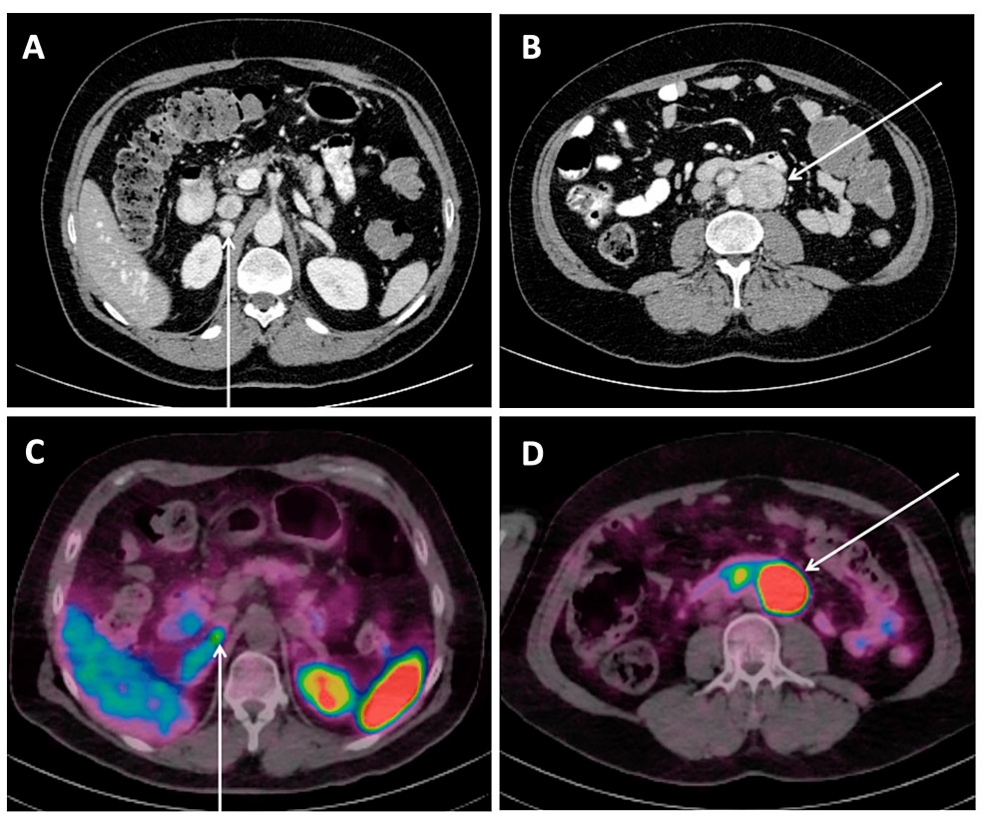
Computed tomography of the abdomen with IV contrast (A, B) and Gallium 68 DOTATATE positron emission tomography CT (C, D) revealing a right adrenal lesion(A, C) and a conglomerate of periaortic lesions(B, D) CT abdomen with contrast showing a right adrenal enhancing lesion measuring 1.4 x 1.2 cm, 190 Hounsfield units post-contrast (A, arrow), and a conglomerate of heterogeneously enhancing periaortic lesions measuring up to 5 cm in the mid-abdomen (B, arrow). In the Gallium 68 DOTATATE PET-CT, a corresponding avid right adrenal nodule (C, arrow) and a retroperitoneal lesion (D, arrow) were demonstrated.
